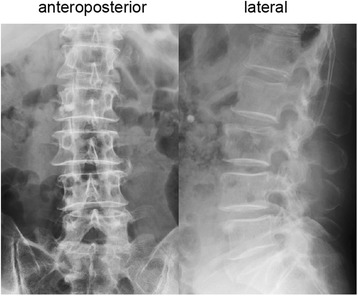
Preoperative radiographs. These are the anteroposterior and the lateral views of the lumbar spine. The preoperative radiographs showed slight wedging deformity and degenerative disc changes
